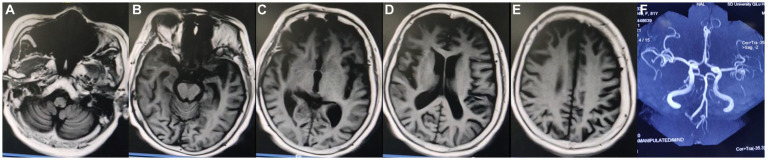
Magnetic resonance (MR) imaging findings dated June 2021. (A–E) Axial MR images showing mild atrophy of the cerebral cortex and thickening of the cerebral dura mater. (F) MR angiography image showing no abnormalities or stenosis in the large intracranial arteries.
